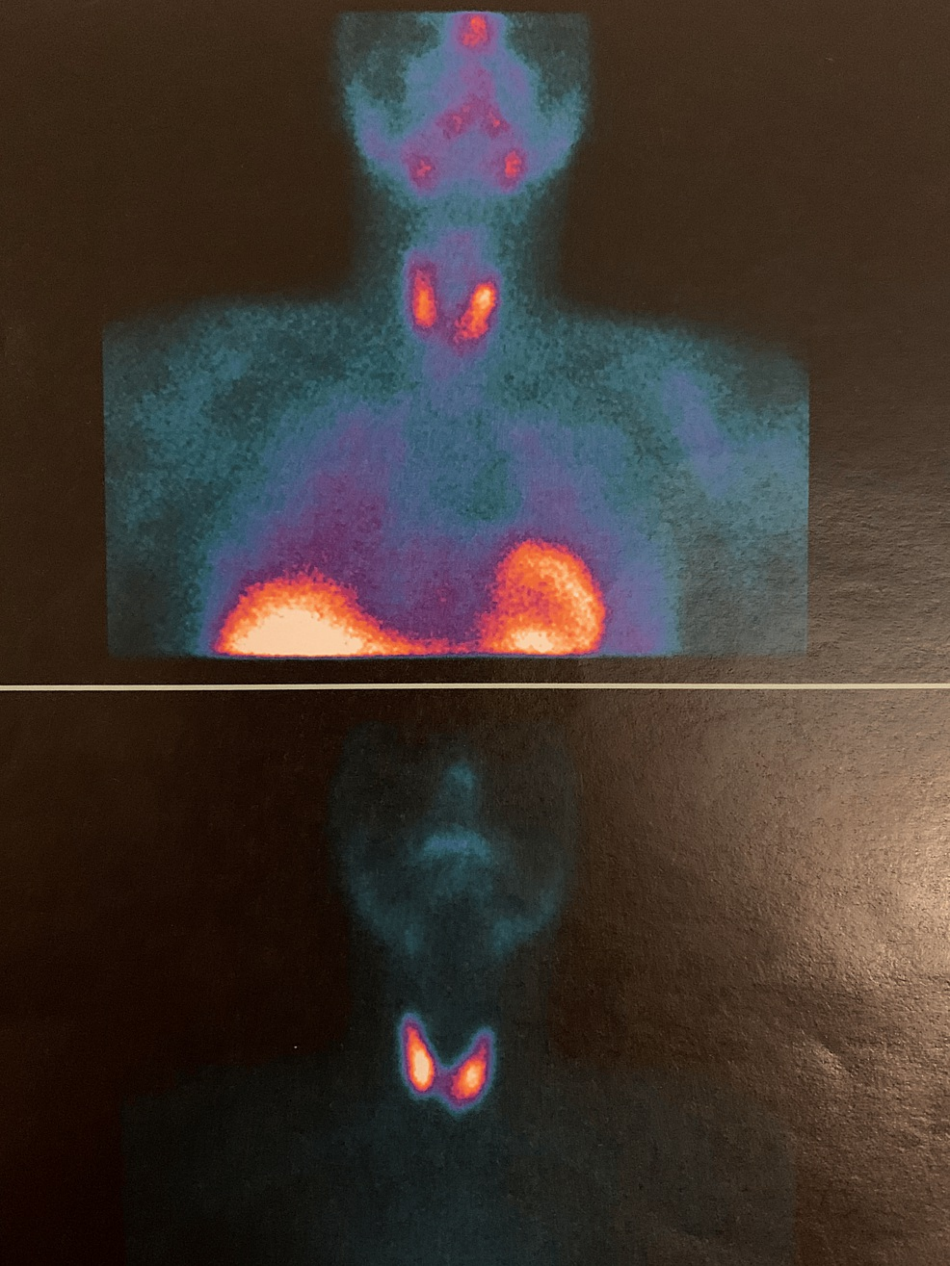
Parathyroid scintigraphy
